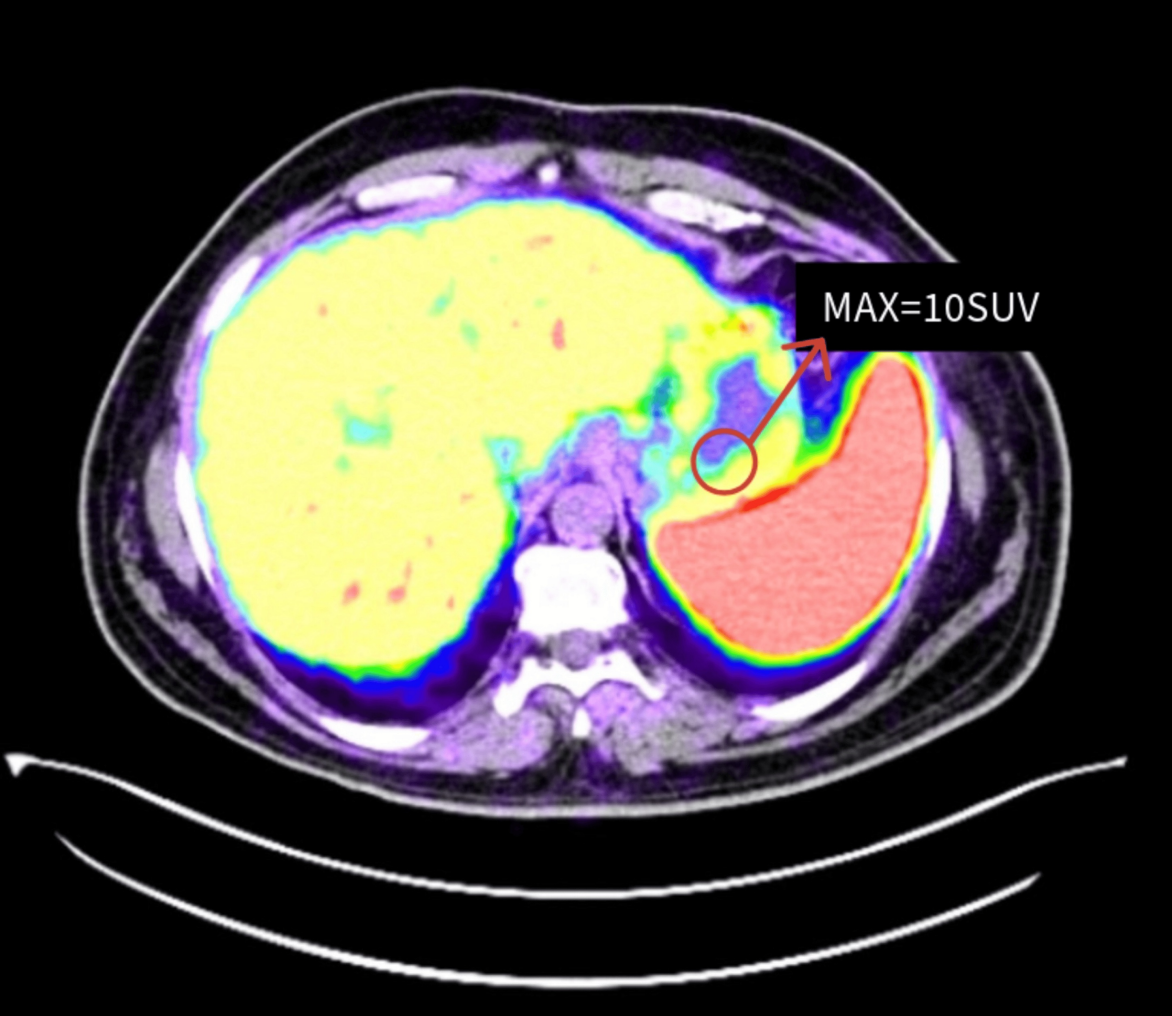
PET-DOTATATE scan revealing a metabolically active lesion with enhanced uptake indicating the presence of somatostatin receptors, suggestive of neuroendocrine tumor involvement. The PET-DOTATATE scan highlights a hypermetabolic nodule in the stomach with a maximum standardized uptake value (SUV) of 10, indicating increased somatostatin receptor activity, consistent with a gastric neuroendocrine tumor. The nodule is located near the greater curvature of the stomach and shows significant radiotracer uptake, confirming the presence of the neuroendocrine tumor.
